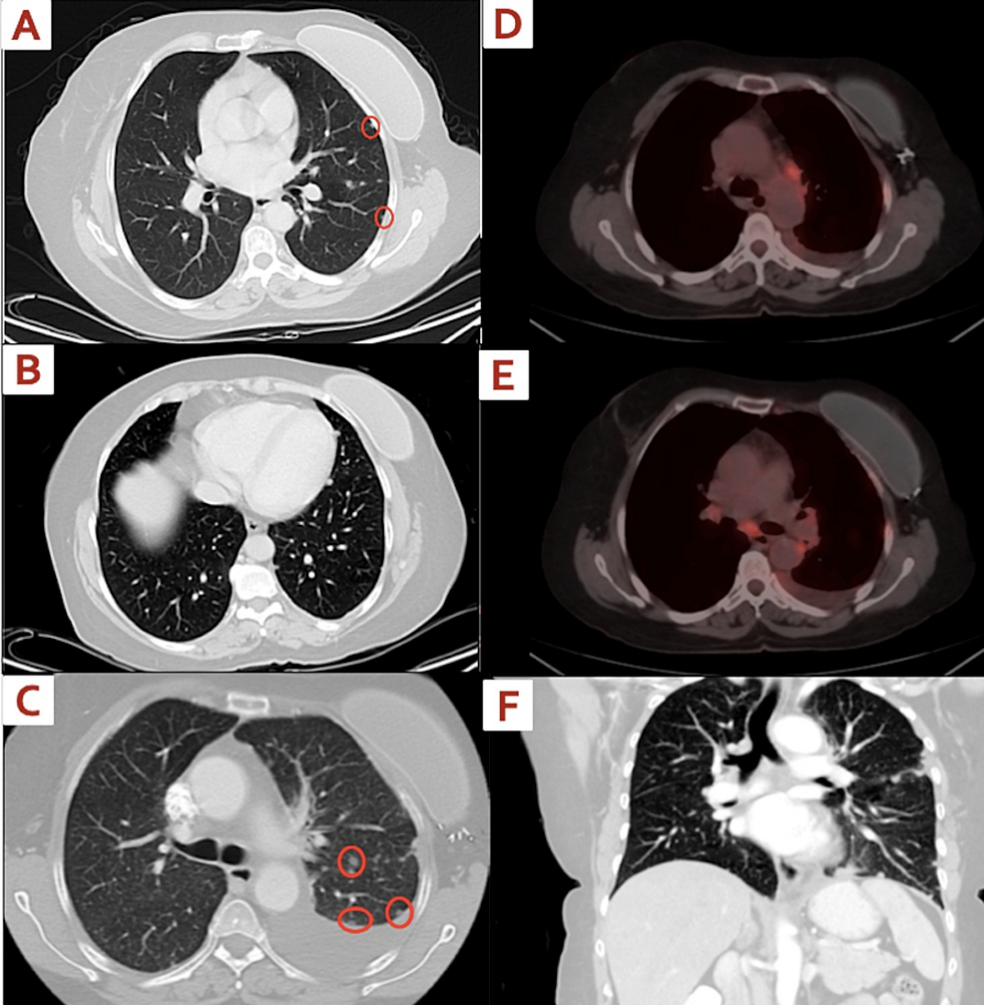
Imaging of nodules (A-C) Chest CT mediastinal window in the transverse plane from April 2016 showing subcentimeter left subpleural lung nodules. (D-E) Chest PET scan with FDG-avid left-sided pleural-based lung nodules. (F) Chest CT scan of the lung window in the coronal plane showing left upper lobe nodule involvement
